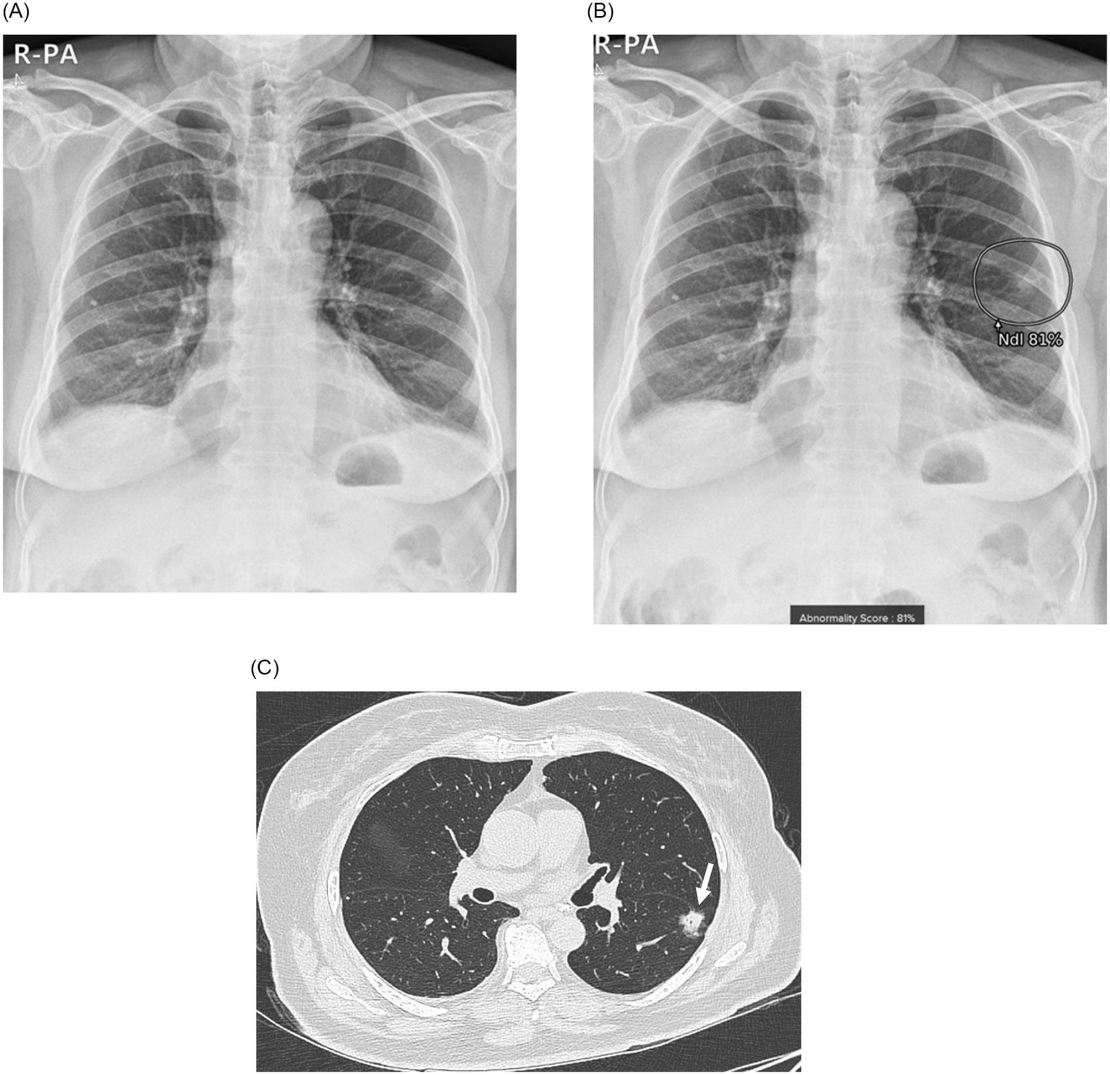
Chest radiograph and computed tomography (CT) of a 65-year-old woman (#6 patient). (A) Chest radiograph was taken at the time of admission to the psychiatric ward for schizophrenia. (B) Artificial intelligence-based lesion detection software detected a lung nodule in the left lower lung field with an abnormality score of 81%. (C) Chest CT demonstrated an irregular solid nodule in the left lower lobe (arrow), and adenocarcinoma of approximately 1.9 cm was confirmed after surgical resection. The final stage was IA2 (pT1bN0M0), and she is now receiving routine follow-up for lung cancer.
